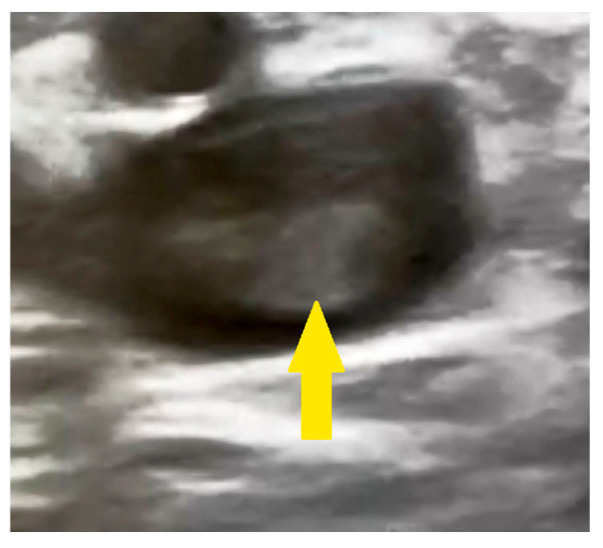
Ultrasound examination of the right calf revealing a thrombus in the popliteal vein (yellow arrow).
